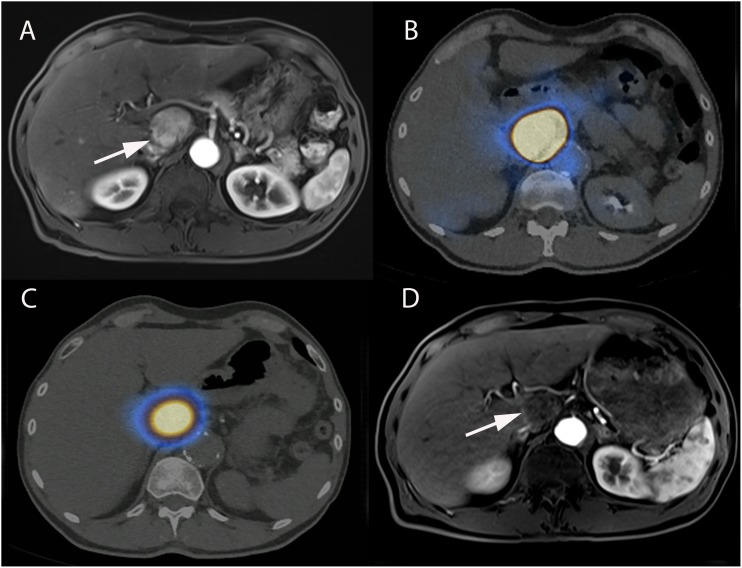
Super-selective SIRT of caudate lobe in a 63-year-old male patient with alcoholic cirrhosis and HCC. (A) Axial contrast-enhanced (arterial phase) T1-weighted MR image showing enhancing tumor in caudate lobe corresponding to HCC (arrow). (B) Pre-treatment SPECT/CT realized after superselective administration of ^99m^Tc-MAA showing activity restricted to caudate lobe. (C) Post-treatment SPECT/CT performed immediately following ^90^Y-microspheres administration, showing excellent superselective tumor targeting and overlap with pretreatment ^99m^Tc-MAA. (D) Axial contrast-enhanced (arterial phase) T1-weighted MR image performed 3-months after SIRT showing a complete response with total disappearance of any intratumoral enhancement (arrow).
